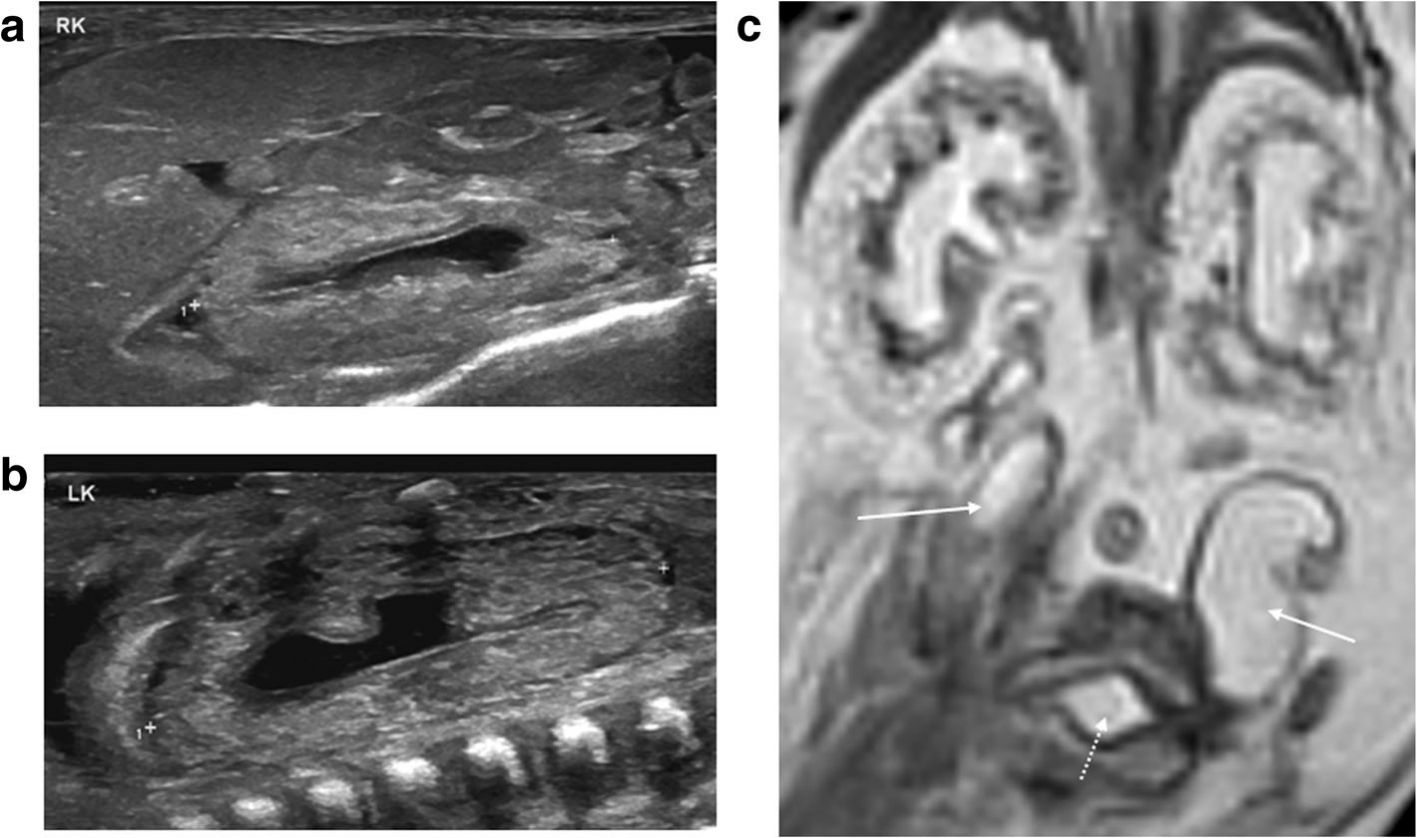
Dysplastic renal appearances in a foetus at 21 weeks gestation, seen as a result of bladder outflow obstruction. Post-mortem imaging was performed 4 days after termination of pregnancy. On the sagittal post-mortem ultrasound images of the right (a) and left (b) kidneys, there is loss of the normal corticomedullary differentiation with hyperechoic renal cortices and mild pelvicalyceal dilatation. The corresponding post-mortem T2 weighted MRI study in coronal plane (c) confirms the same findings as ultrasound, and also reveals bilateral dilated and tortuous ureters (solid arrows) leading to a partially collapsed urinary bladder (dotted arrow)
